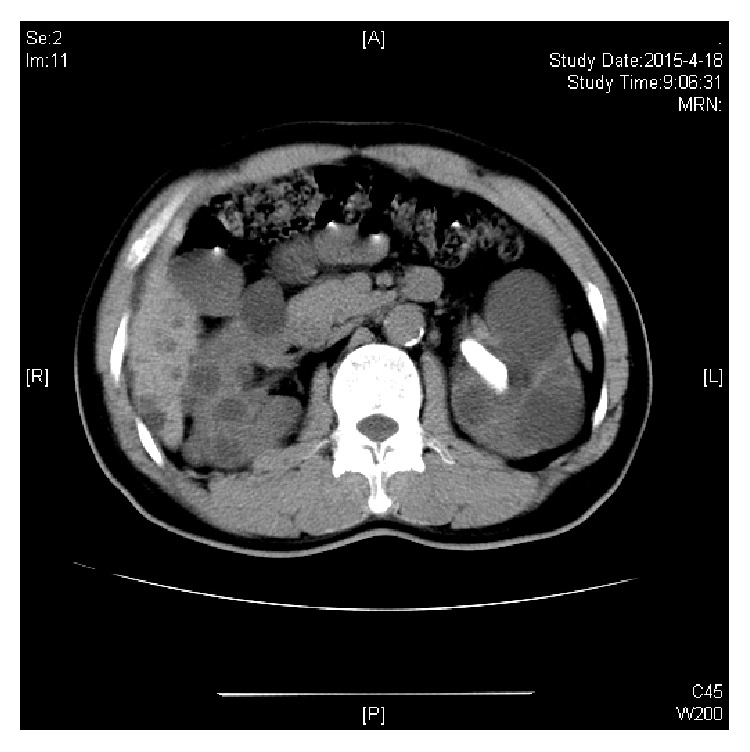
CT scan: left renal calculus with multiple cysts.
